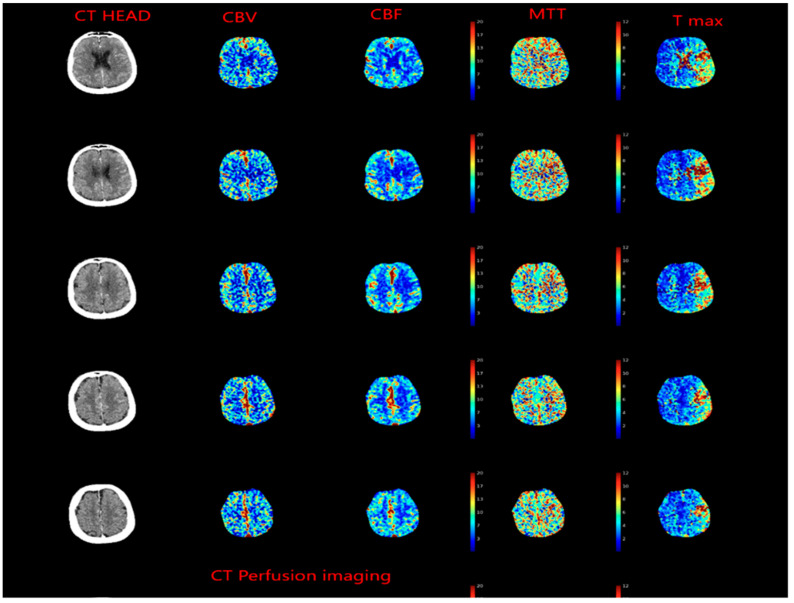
CT Perfusion imaging in patient presenting with left middle cerebral artery occlusion, cerebral blood volume (CBV) is preserved, whereas cerebral blood flow (CBF) is decreased, and mean transit time (MTT) is increased.
